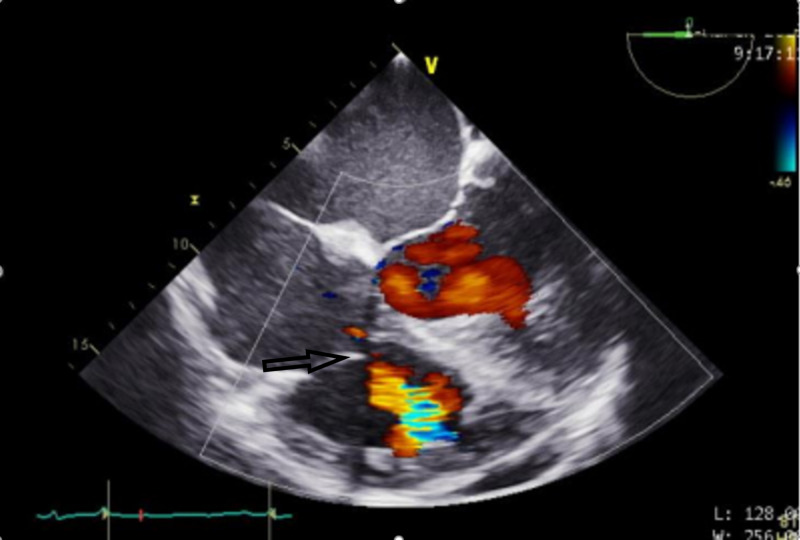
Midesophageal four chamber view. Color flow Doppler is used to show flow through the VSD shown by the arrow. VSD, ventricular septal defect
